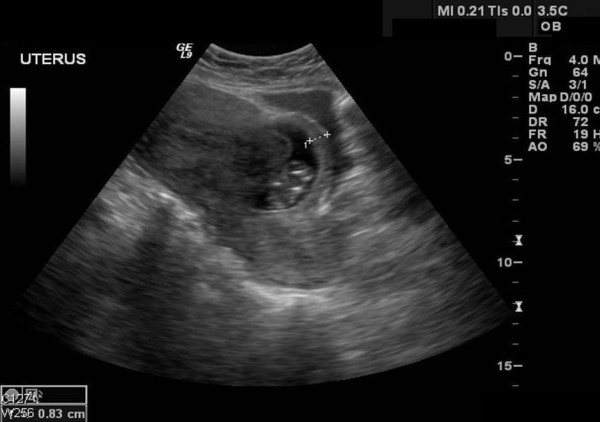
Abdominal ultrasound, longitudinal scan showing a gestational sac pushing itself towards the urinary bladder. An 8 mm layer of fibro-muscular tissue separates the sac from the bladder.
